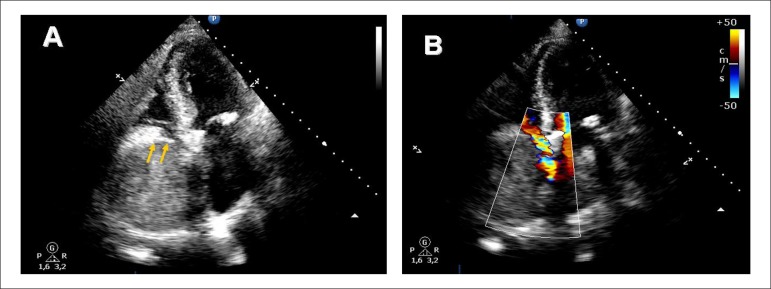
Transthoracic echocardiography: giant eco-dense mass occupying the entire right
